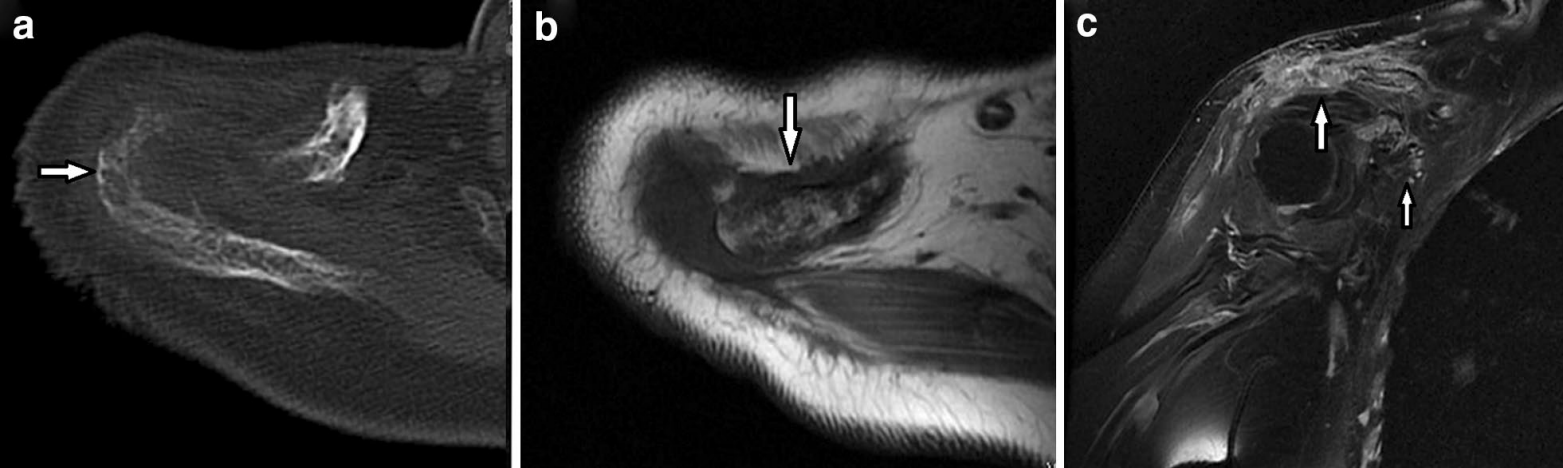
Case 7: a Unenhanced CT image shows irregularly bone destruction (*white arrow*). b, c Axial T1-weighted MR image and coronal fat-supressed T1-weighted postcontrast image show multiple, ill-defined lesions of the acromioclavicular joints and coracoid (*white arrow*) with geographically pattern and heterogeneous enhancement
